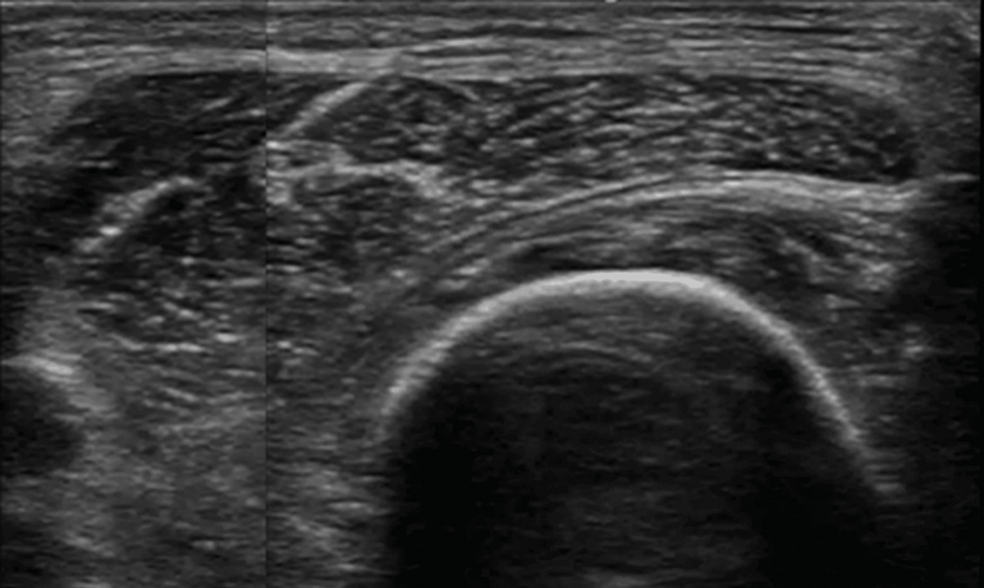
Representative ultrasound image of a non-uniform muscle cross-section of the rectus femoris. The image was created by combining two separate images.
